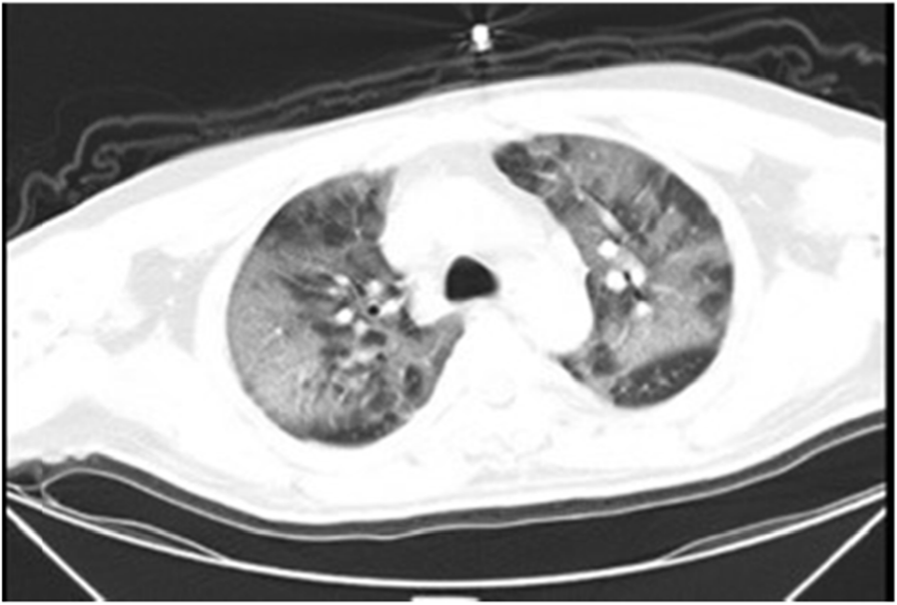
CT imaging of case 1 infected with SARS-CoV-2 on January 5, 2020, Illness day 7
